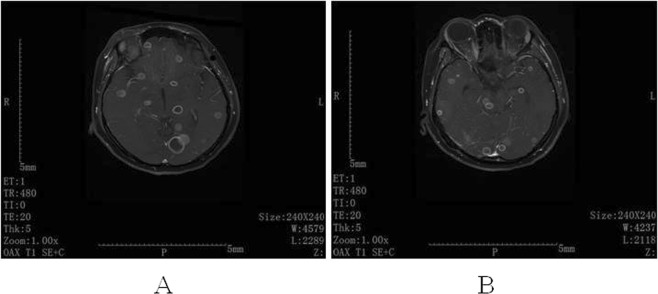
Cerebral enhanced MRI of case 17. (A) Multiple nodular and annular enhanced lesions in cerebrum and cerebellum. (B) Multiple nodular and annular enhanced lesions in cerebrum, cerebellum and brainstem.
